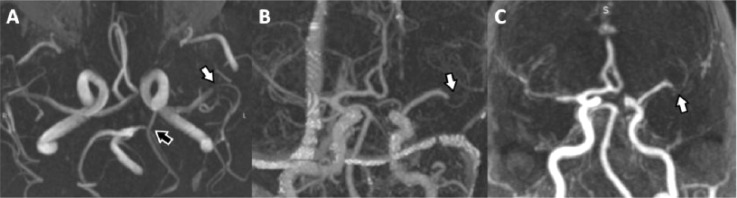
Case 2 - Partial occlusion of the majority of the left MCA-M2-branches was already known from MRA performed several years before the reported acute event (A*, axial view, white arrow*). Acutely performed CTA (B*, white arrow*) and MRA (C*, white arrow*) confirmed this finding. Note that an initially visible fPCA (A*, axial view, open black arrow*) was no longer visible in the acutely performed examinations. CTA, CT-angiography; fPCA, fetal posterior cerebral artery; MCA, middle cerebral artery; MRA, MR angiography.
